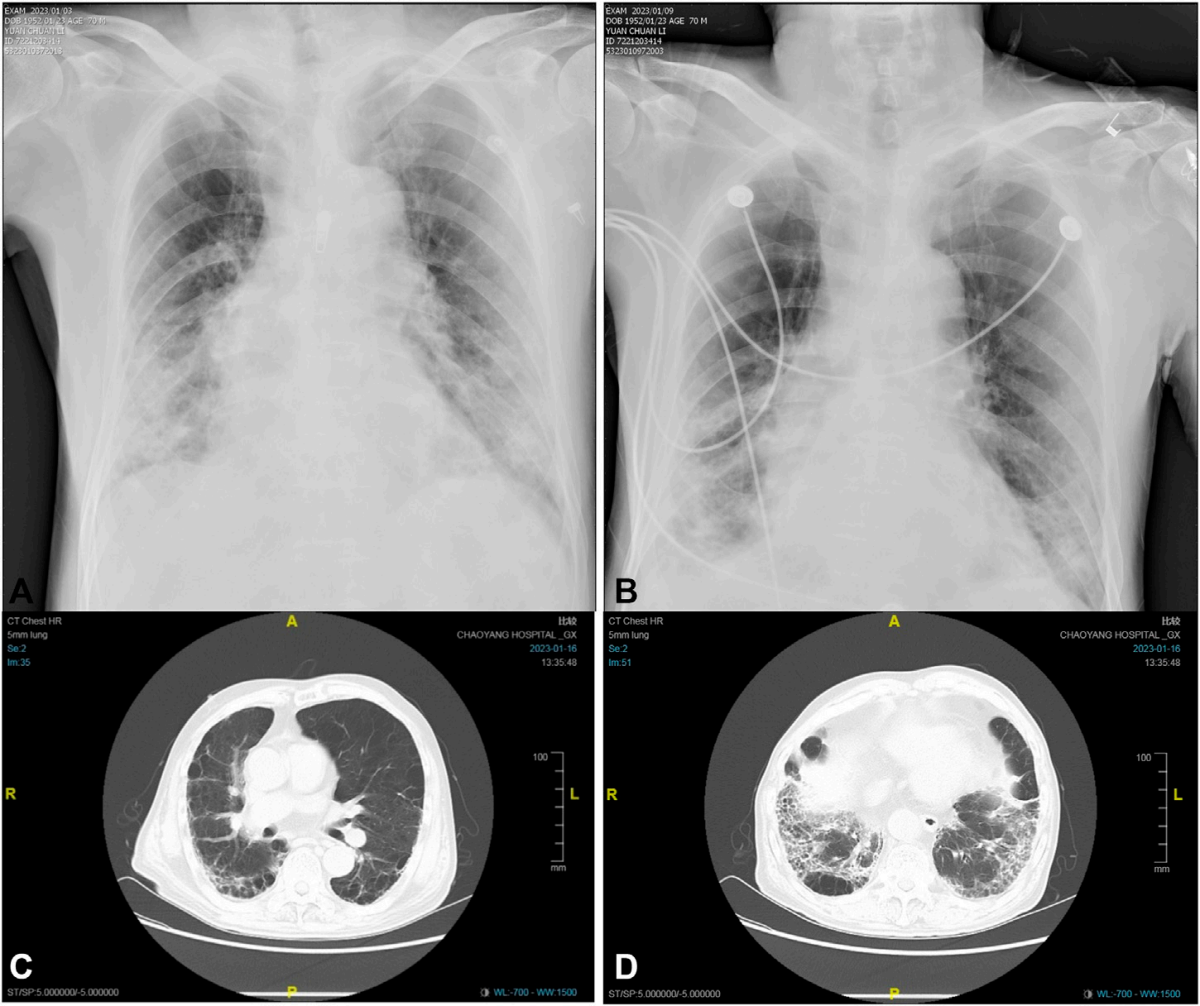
Dynamic chest X-ray and CT images of the patient:(A) the X-ray image on day 9, (B) the X-ray image on day 15, (C) the CT image on day 22, and (D) the CT image on day 22.
